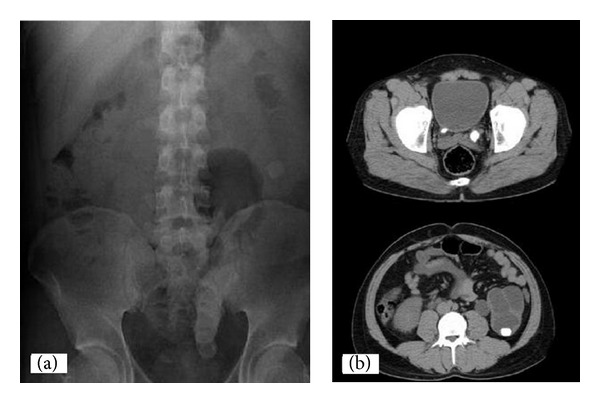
Plain film shows a long radiopaque density (11.5 cm) suggesting a giant ureteral stone in the pelvic area (a). The CT scan of the abdomen shows a left giant ureteral stone measuring 11.5 cm causing ureteral obstruction and other stones 2.5 cm in size in the lower pole of ipsilateral kidney and 7 mm in size in distal part of right ureter (b).
